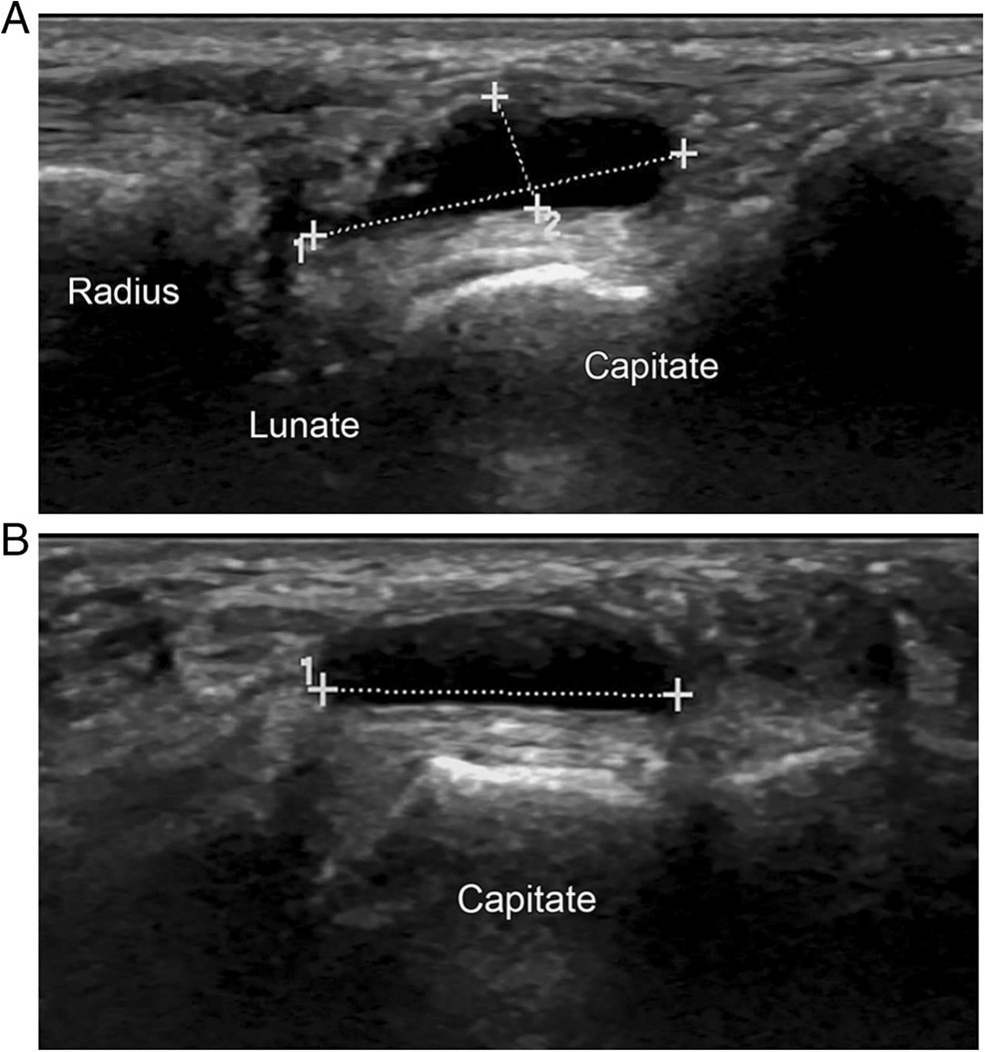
Dorsal ganglion cyst. Ultrasound images of dorsal wrist in (A) sagittal and (B) transverse planes show anechoic multilobular ganglion cyst (between cursors). Right side of image A is distal (Zhang, 2018). Reprinted with permission from Wiley Online Library [41]
